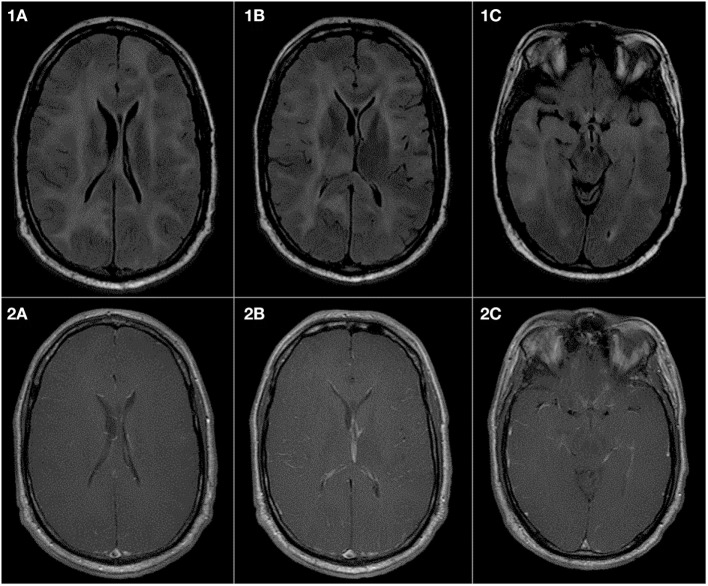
Gliomatosis cerebri presenting in a 43-year-old man as a syncopal episode, insidious onset of slurred speech and left leg weakness. Pathology was consistent with IDH-wildtype anaplastic astrocytoma. (1A–1C) are FLAIR sequences demonstrating extensive abnormalities in the white matter of both cerebral hemispheres presenting as high signal intensity. There is also an involvement of midbrain and the right thalamus. The right temporal lobe shows more extensive abnormality as compared to the left temporal lobe. (2A–2C) are T1 sequences postcontrast and show no abnormal increased enhancement in the brain parenchyma or in the meninges.
